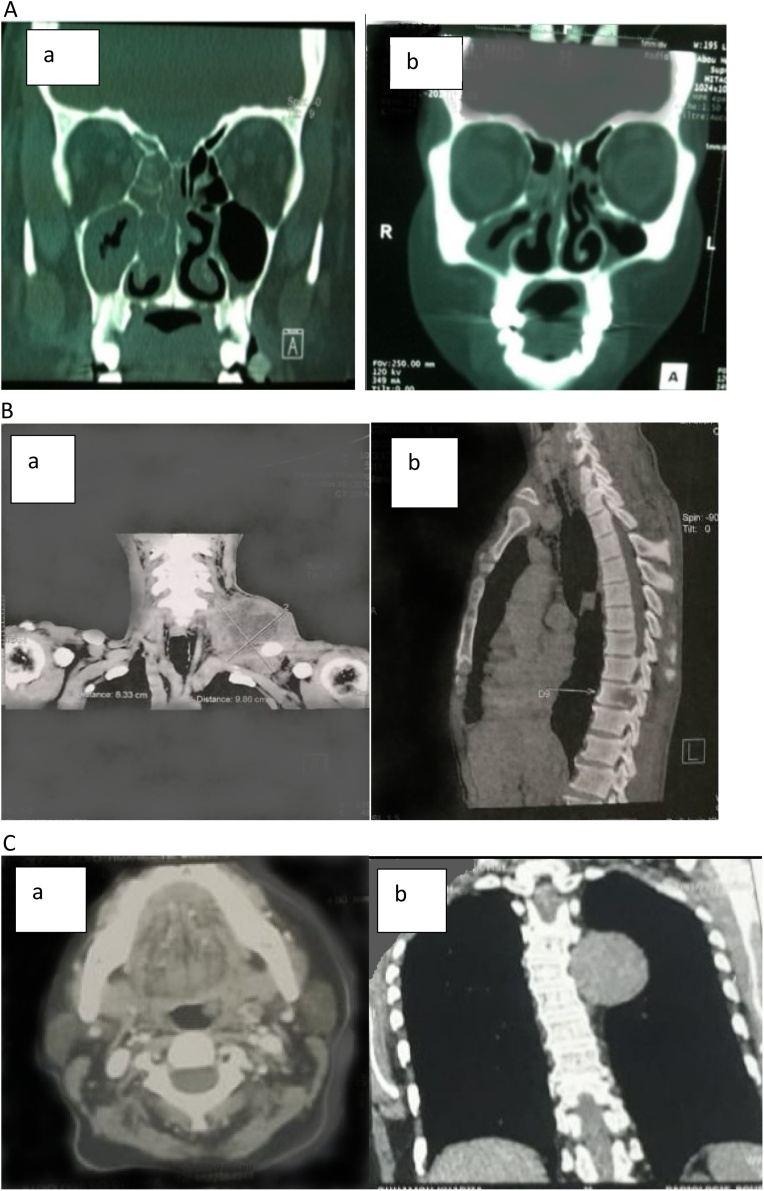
A: Facia CT scan: heterogeneous mass filling the right nasal cavity, maxillary sinu with bone destruction of the right nasal walls. (a): Before chemotherapy and radiotherapy. (b): After chemotherapy and radiotherapy (regression of the tumor). B: cervical CT scan: (a): Left compressive basi cervical mass. (b): Dorsal vertebral bone lysis. C: Cervical + Chest CT scan: (a): Mass of the left tonsil. (b): Left posterior mediastinal mass.
